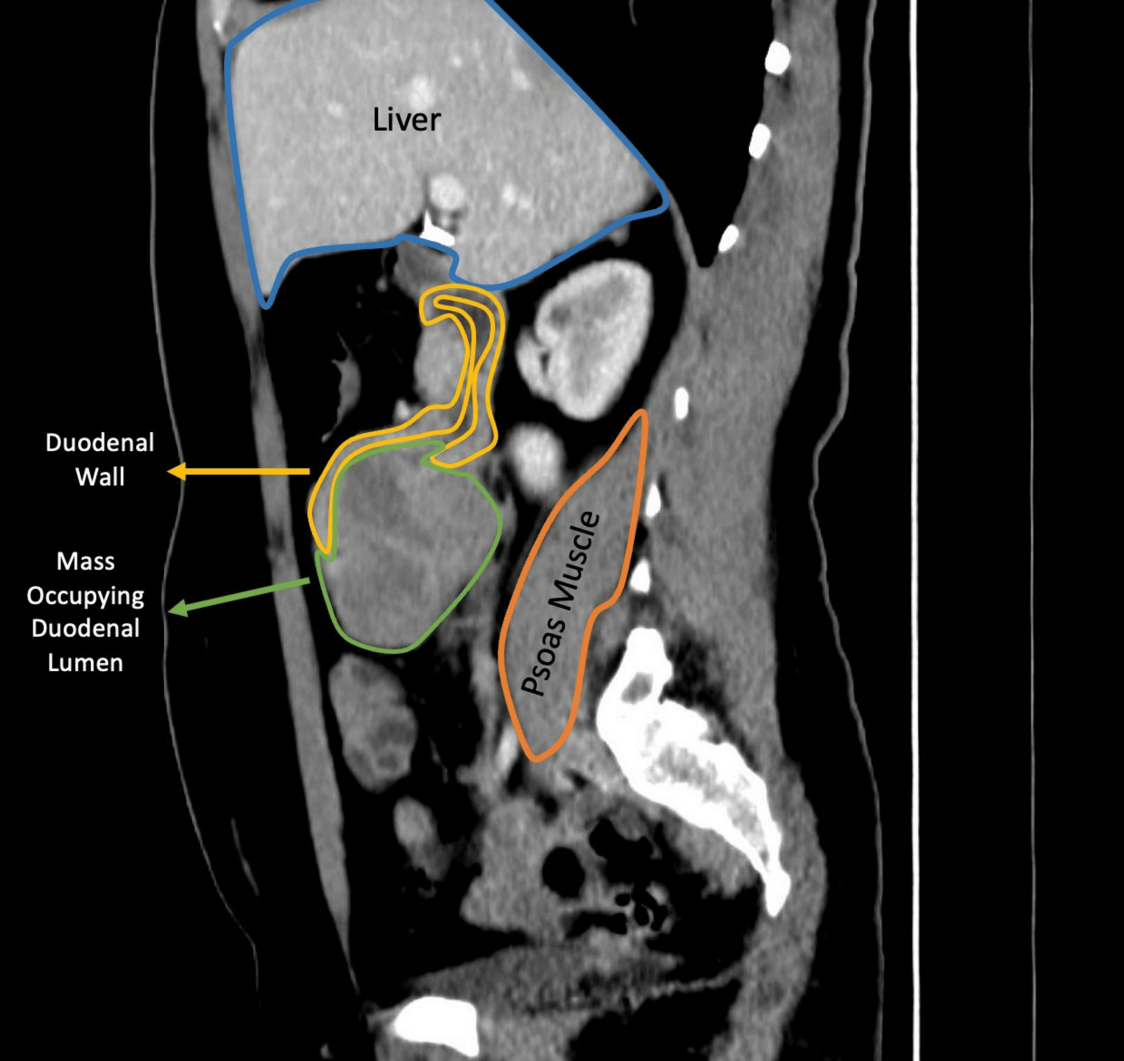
Contrast-enhanced sagittal CT image of a polypoid intraluminal duodenal mass A large heterogeneously enhancing intraluminal duodenal mass is seen. The lesion projects into the lumen in a polypoid fashion from the posterior wall, and its proximal margin forms an acute angulation with the duodenal wall.
